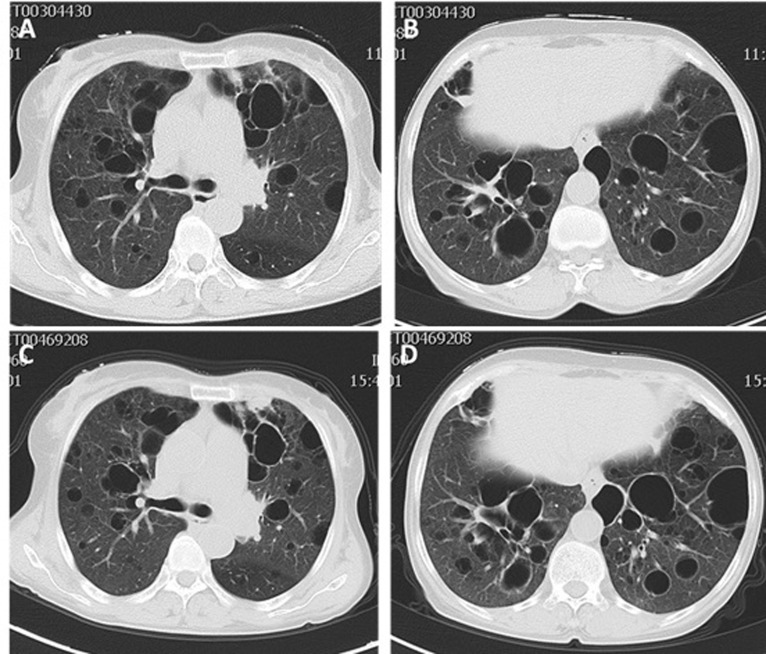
A.-B. Chest high resolution computed tomography (HRCT) images in 2014 showing bilateral diffuse thin walled cystic changes with a wide variation in cyst size and distribution. C.-D. Chest HRCT images in 2016 which were almost unchanged from 2014.
